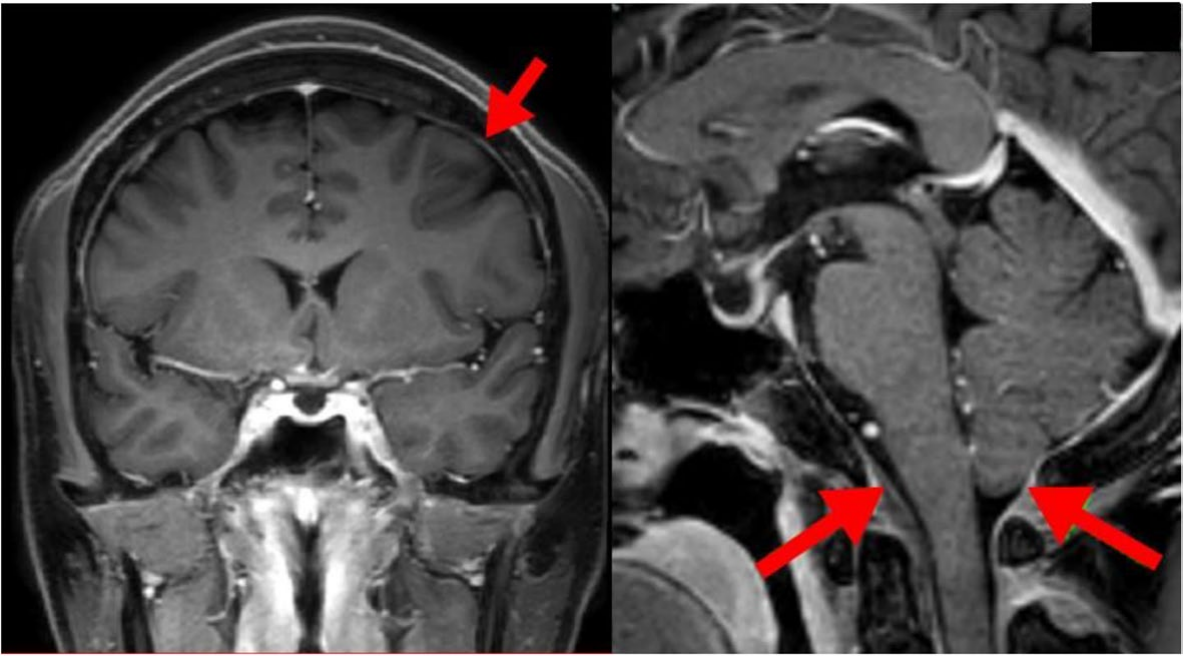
Follow-up brain MRI results. Brain sagging and dural thickening improved after treatment (arrows)
